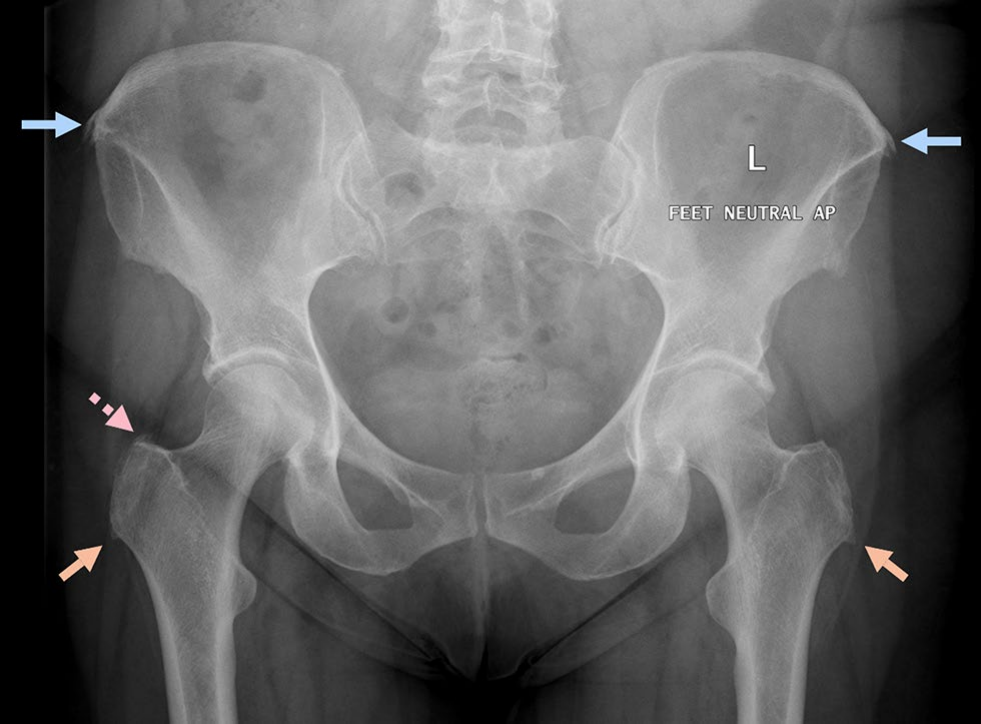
Tendon calcifications evident on radiographs. Superior/Blue arrows: Gluteus medius origin at iliac crest. Dotted/ Pink arrow: Gluteal tendons at the superolateral aspect of the greater trochanter. Inferior/Orange arrows: Calcifications extending inferiorly from the greater trochanter
